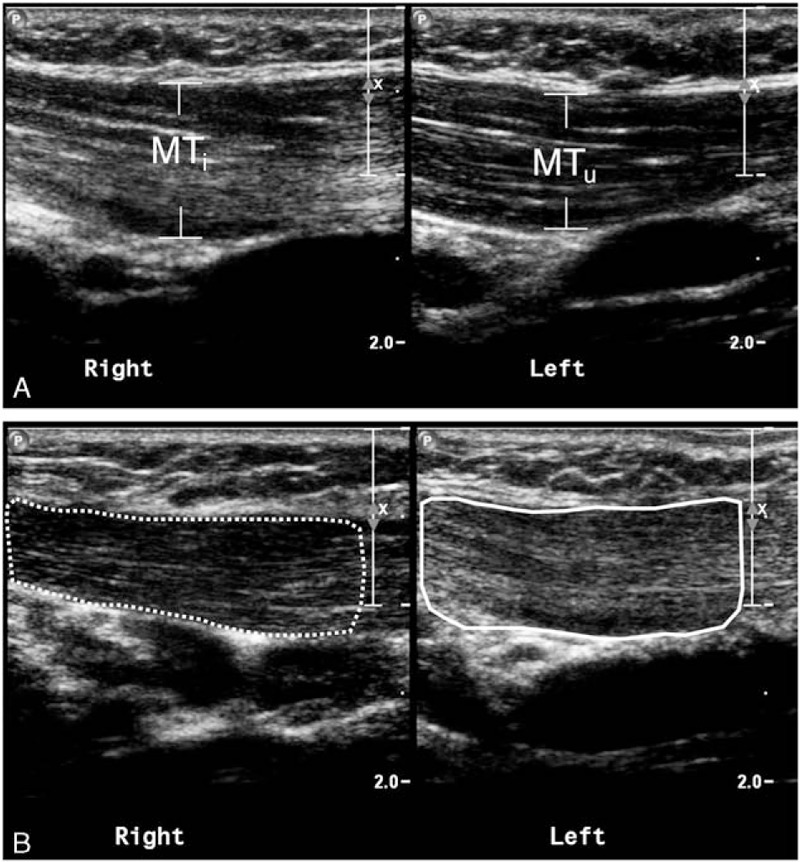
US of bilateral SCM muscles in infants with CMT. (A) Longitudinal sonograms of maximum anterior–posterior diameters of the involved (MT_i_) and uninvolved (MT_u_) SCM muscles. Ratio I/U was calculated as MT_i_/MT_u_. (B) Longitudinal sonograms of the *MEI* of every pixel in the ROI in the uninvolved (dotted line) and involved (solid line) muscles. The *K* value was calculated by *MEI*_uninvolved_ − *MEI*_involved_.
